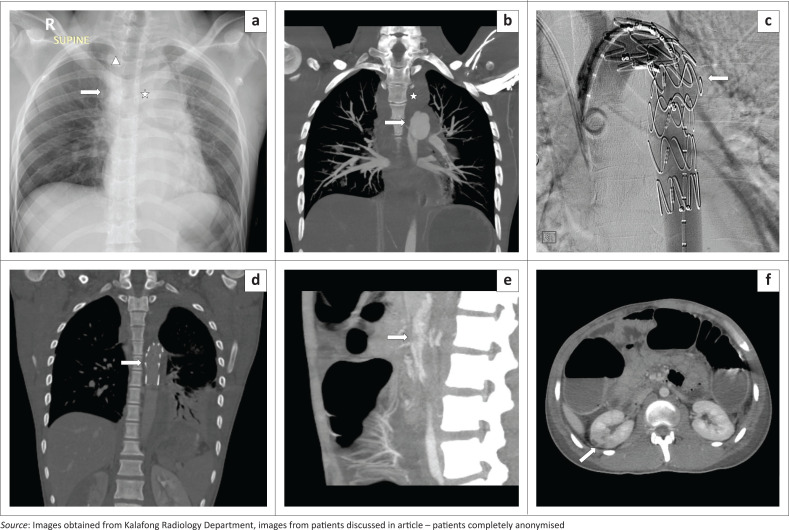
(a) Chest radiography in a 23-year-old male involved in a pedestrian vehicle accident indicates a widened mediastinum (arrow), loss of the aortic knuckle (star), retro-cardiac double density and a widened right paraspinal line (triangle). (b) Coronal computed tomography angiography image with a type 2 aortic injury, complicated by thrombus formation (arrow). Also note the large mediastinal haematoma (star). (c) Sagittal digital subtraction angiogram after endovascular aneurysm repair with the stent in place (arrow). (d) Follow-up computed tomography angiography post stenting. (e) Sagittal computed tomography angiography abdomen demonstrating subtle thrombus within the coeliac artery (arrow). (f) Segmental infarction revealed within the right kidney (arrow).
